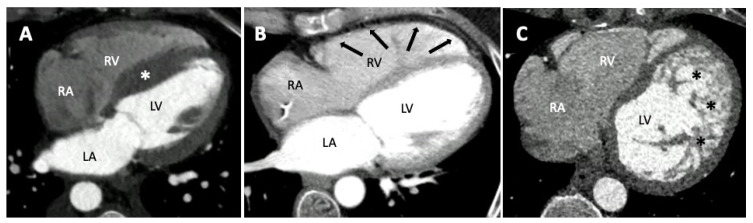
Cardiovascular computed tomography imaging presenting three patients with different cardiomyopathy. Panel (A): Hypertrophic Cardiomyopathy (HCM). The white asterisk indicates the hypertrophic septum. Panel (B): Arrhythmogenic cardiomyopathy right. The black arrows indicate the fibrofatty deposition of the right ventricle in the dual-energy CCT. Panel (C): Left ventricular non-compaction. The black asterisks indicate the prominent trabeculae of the left ventricle. Right ventricle (RV); left ventricle (LV); right atrium (RA); left atrium (LA).
